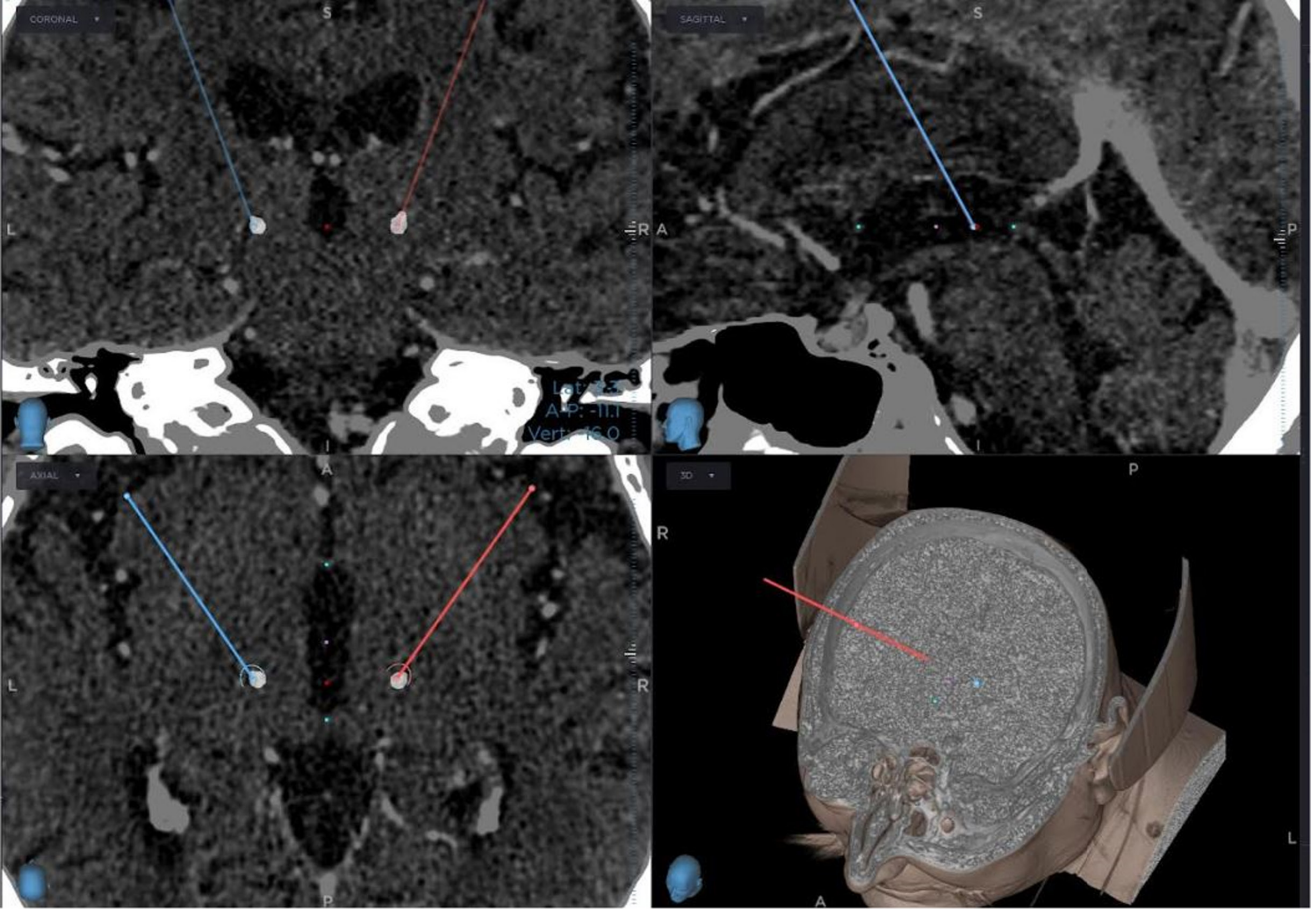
Co‐registration of preoperative CT with contrast media and postoperative CT. Electrodes targeting the Vim are shown in blue (left) and red (right) in the coronal (top left), sagittal (top right), axial (bottom left), and 3d (bottom right) views. The silver circles in the coronal and axial views mark the actual placement of the electrodes. In the sagittal and axial views, the anterior and posterior commissures can be seen (light blue) as well as the MCP (pink).
